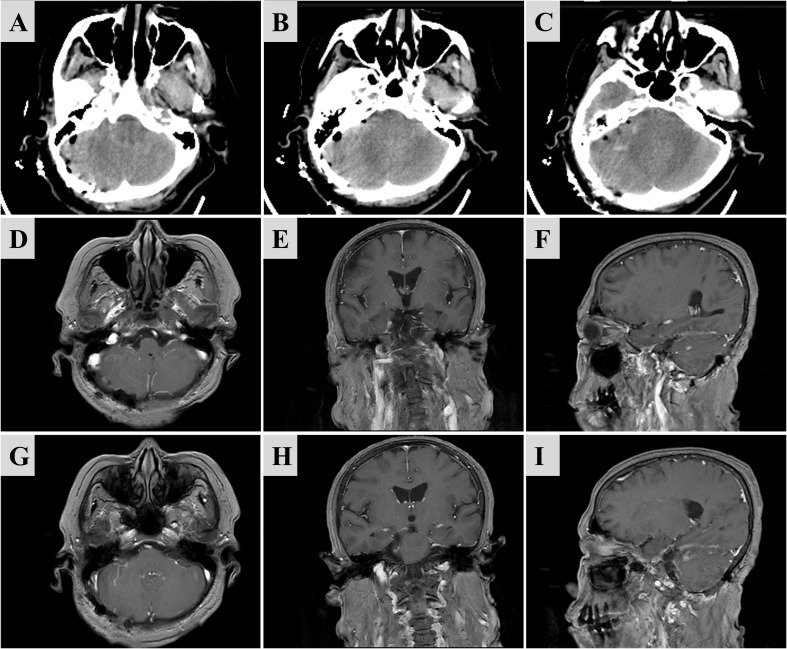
Immediate postoperative CT demonstrated gross total resection of the tumor (A–C). Postoperative contrast-enhanced MRI at follow-up showing gross total resection of the tumor (D–I).
